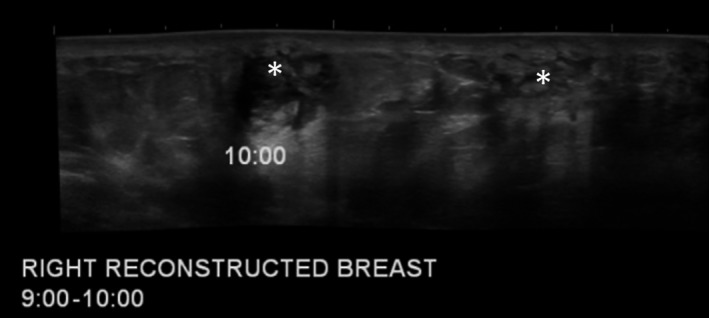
Representative ultrasonographic evaluation at the time of digital mammogram of the Z3 region of the right reconstructed breast (Figure 2) demonstrating mixed echogenic masses (*) at the 9‐10:00 position
